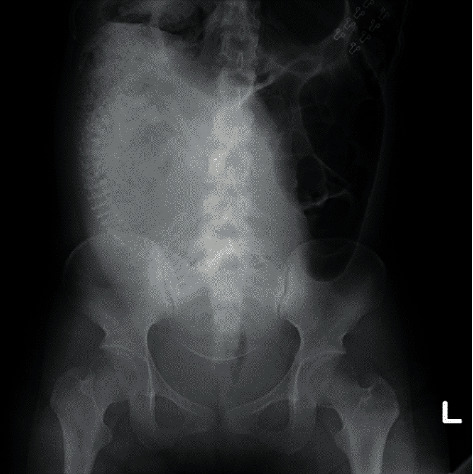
Plain abdominal radiograph showing left-sided dilated bowel.
